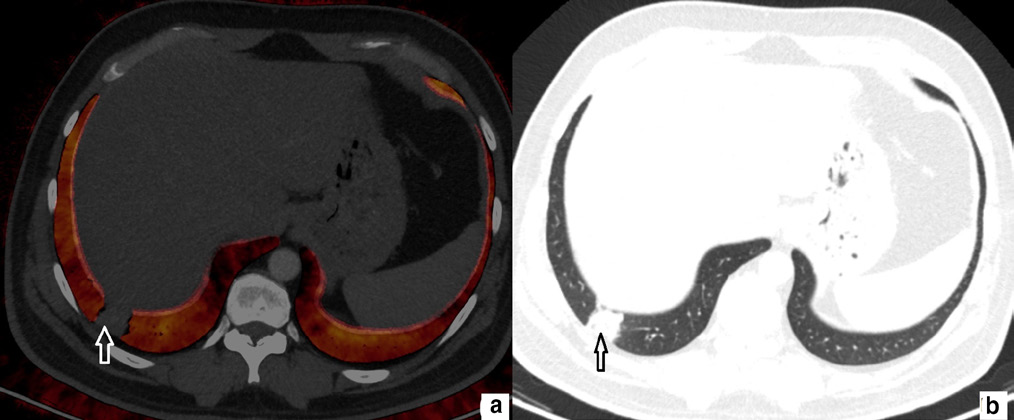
52-year-old female. Axial perfusion blood volume (a) and computed tomography (b) images. Focal consolidation at posterior basal segment (b, arrow), matching perfusion deficit is present on perfusion blood volume image (a, arrow).
